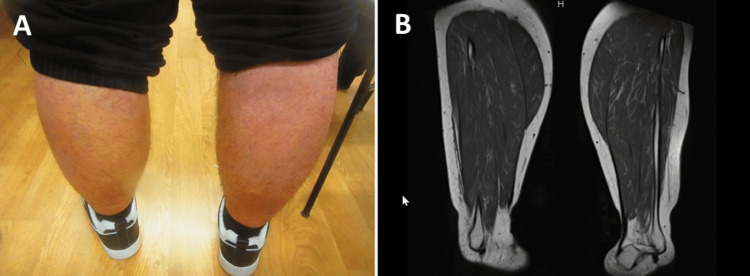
Dwarf calves without fatty or fibrous degeneration on assessment (panel A) and on muscle MRI (panel B) MRI: magnetic resonance imaging
